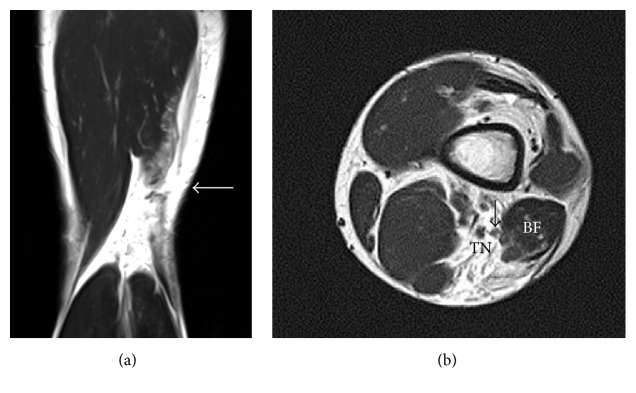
T2-weighted coronal magnetic resonance image of left thigh. Complete rupture of distal biceps femoris tendon at the musculotendinous junction (a). T2-weighted axial magnetic resonance image of left thigh shows close relationship between common peroneal nerve (arrow) and biceps femoris muscle (b). BF, biceps femoris muscle; TN, tibial nerve.
